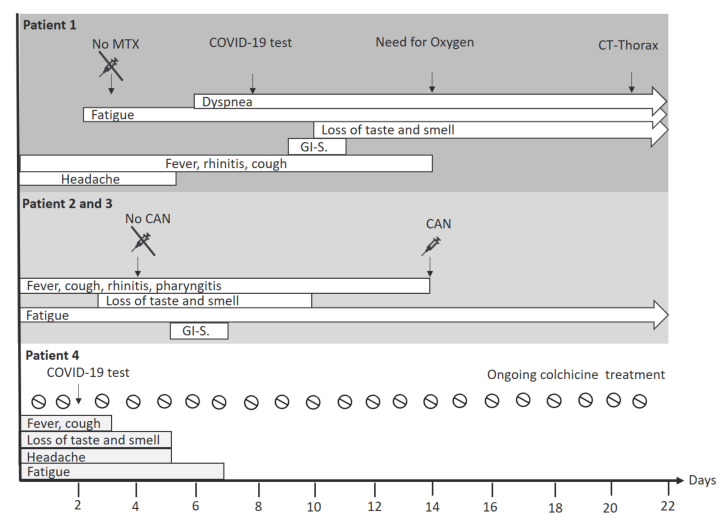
Overview of symptoms of the acute COVID-19 disease course in AID patients. Acute COVID-19 disease course was less severe in the three adolescent AID patients with canakinumab maintenance treatment as compared to the adult patient treated with methotrexate. Particularly patient 4, who was treated with daily colchicine and who had received canakinumab 150 mg s.c. 4 days before COVID-19 was confirmed, had a short and mild disease course. Abbreviations: CAN: Canakinumab; CT: Computed tomography; COVID-19: Coronavirus disease 2019; GI-S.: Gastrointestinal symptoms such as nausea, diarrhea, emesis, abdominal pain; MTX: Methotrexate. Legend: ⇨ ongoing disease symptoms on day 22 after COVID-19 onset.
